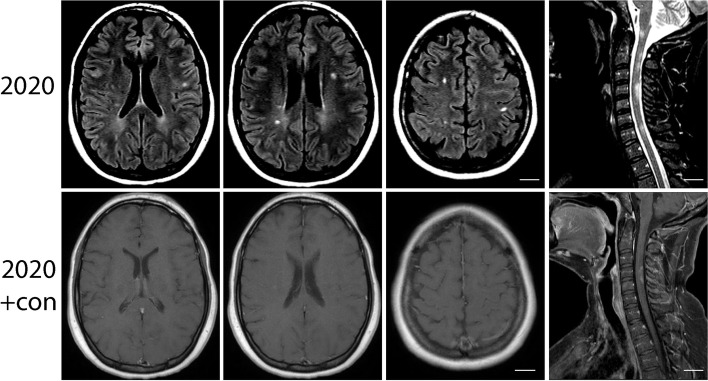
T2-weighted FLAIR MRI of the brain showing subcortical and periventricular lesions in case 2. STIR-weighted image of the spinal cord showing multiple cervical lesions. A subset of these lesions were enhancing (row 2)
